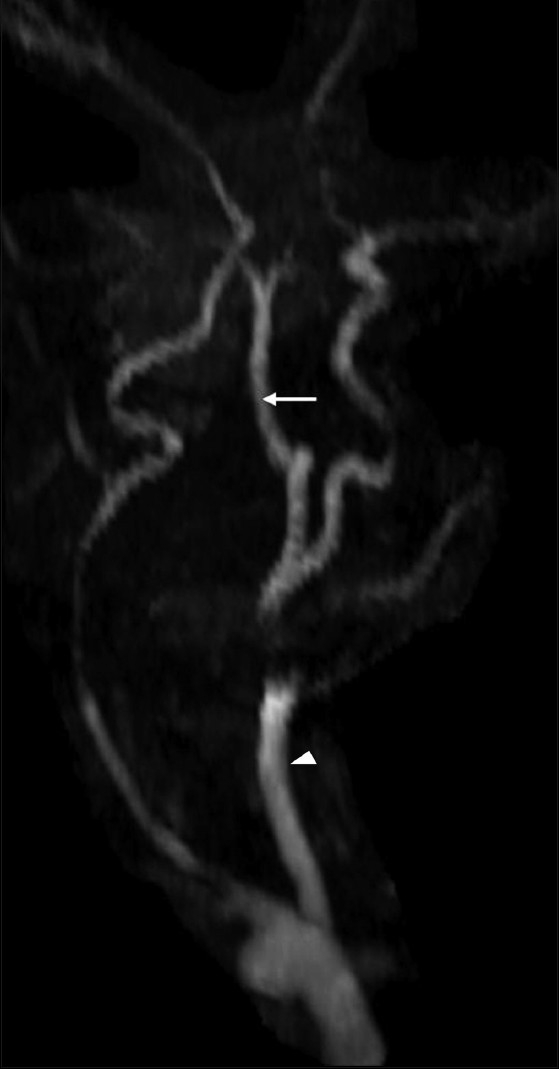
Time of flight MRI angiography shows absence of both vertebral arteries with a persistent primitive hypoglossal artery (white arrow) arising from the left internal carotid artery (arrowhead)
